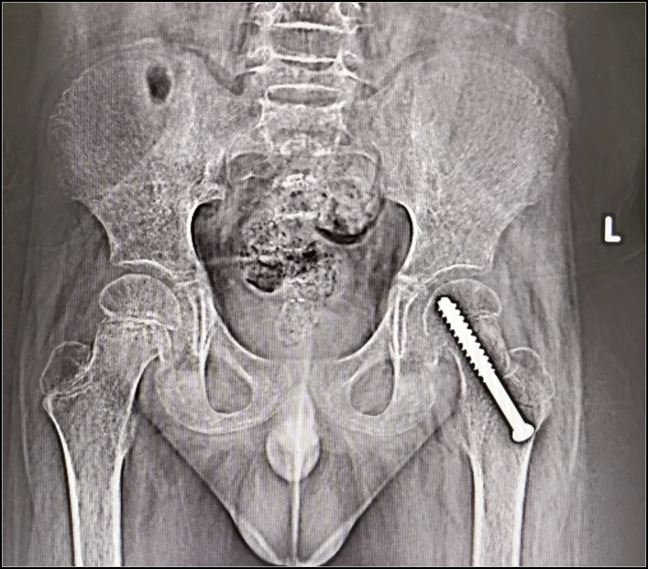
Radiograph at the 6-month follow-up showing screw in situ and no evidence of slip progression and osteonecrosis.
